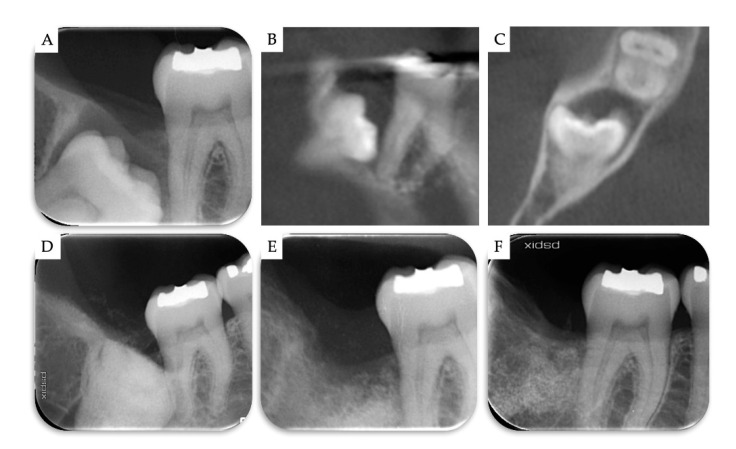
Case no 1. (A–C) Periapical X-ray and CBCT images at T0. Periapical X-rays (D) immediately after the removal of M3 and grafting with β-TCP/CS, (E) 4 months post-operatively, and (F) 2 years post-operatively.
